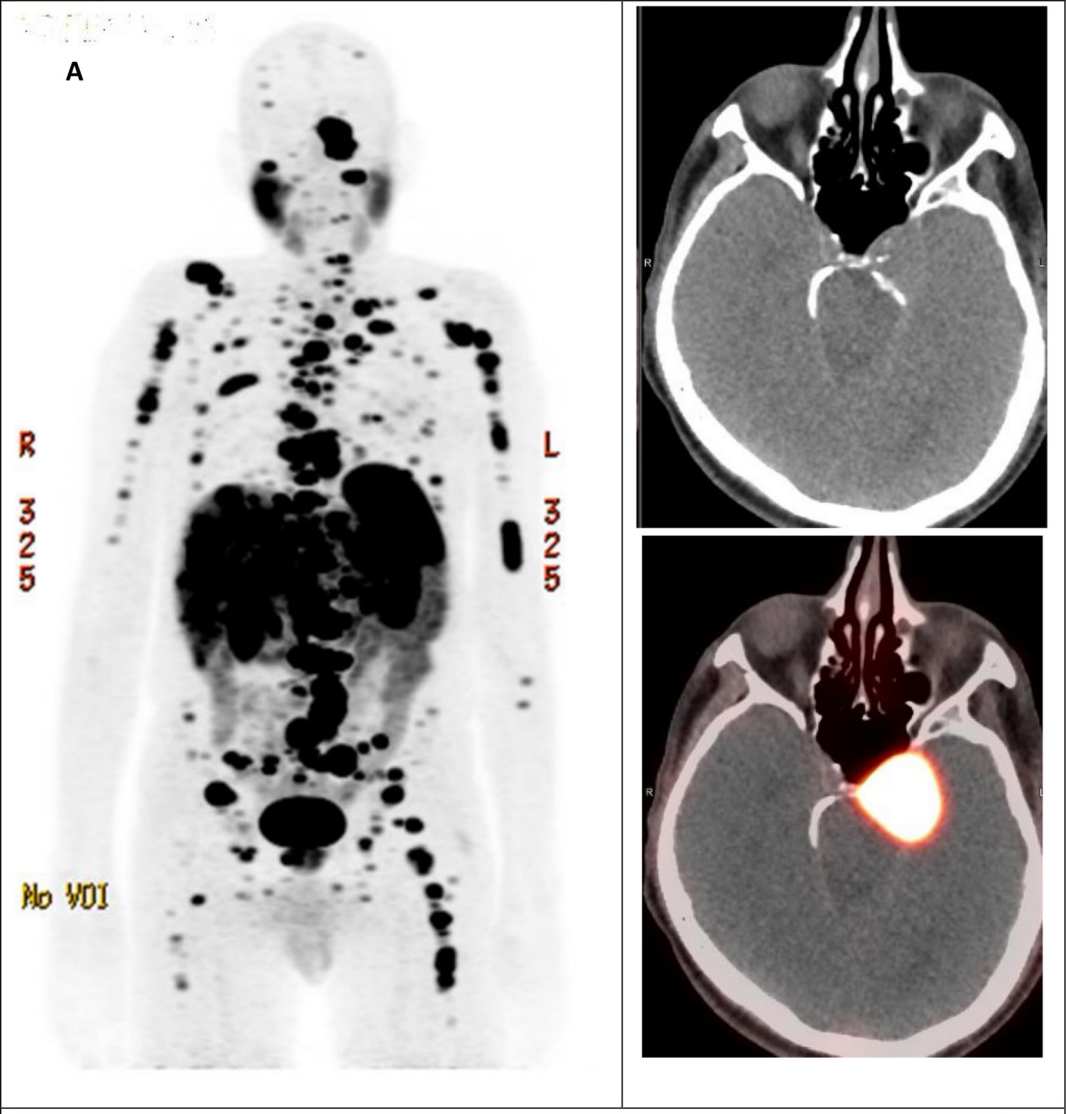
A. Gallium68-DOTATATE PET/CT MIP image shows numerous tracer avid lesions involving the skeleton, lymph nodes and liver, compatible with somatostatin receptor positive lesions. (B, C) The residual left skull base lesion demonstrates intense tracer uptake with SUVmax 29.0. MIP: Maximal Intensity Projection. SUV: Standard Uptake Value.
